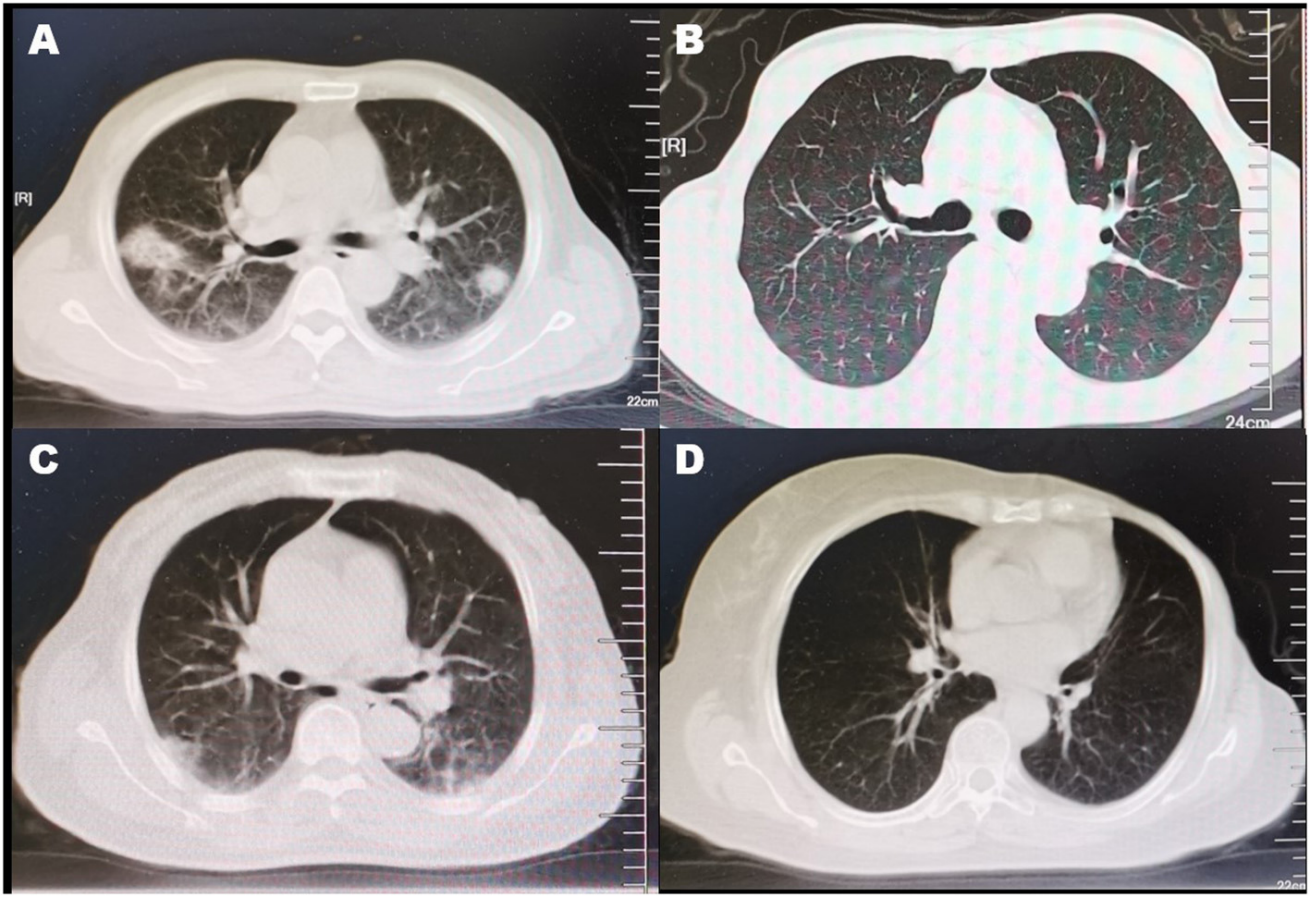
CT findings of COVID-19 patients. (A) Enlargement of bronchi and vascular and mixed consolidation and GGO were found in this symptomatic COVID-19 with pneumonia patient. (B) There was absence of abnormality in CT scan of this symptomatic COVID-19 without pneumonia patient. (C) Although this asymptomatic pneumonia patient had absence of symptoms, the CT scan found enlargement of bronchi and vascular and subpleural GGO and band. (D) This asymptomatic COVID-19 without pneumonia patient was observed with normal radiographic presentation in CT scan.
